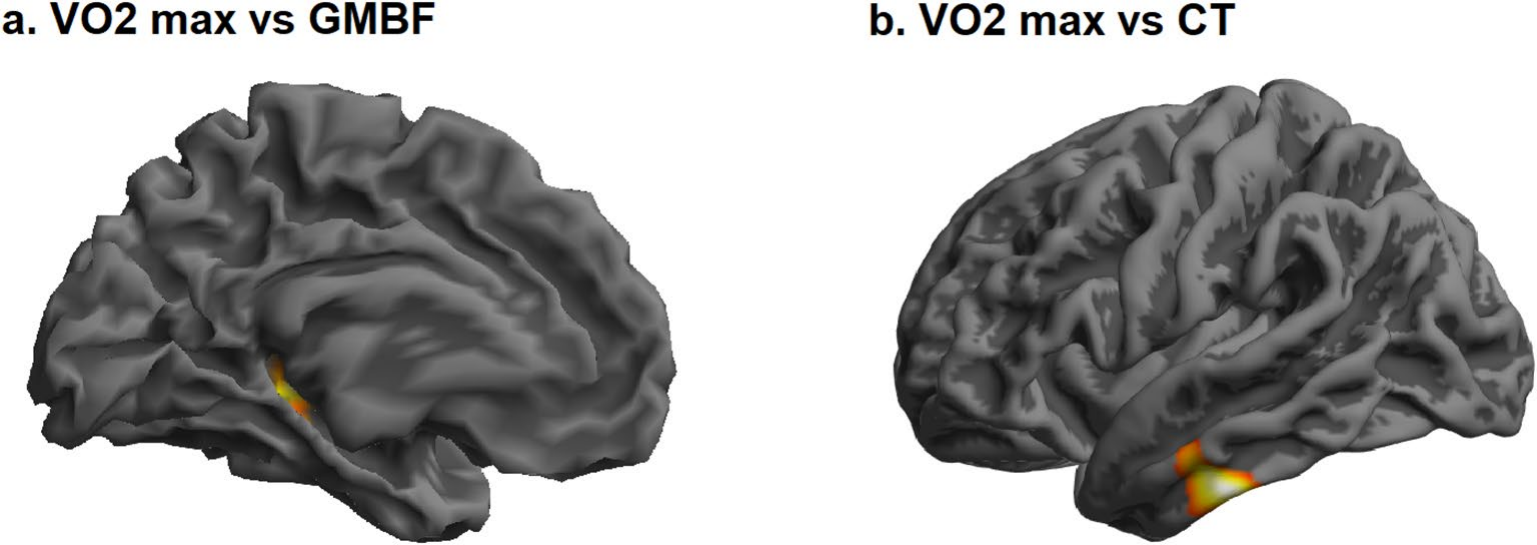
Association between VO_2_max and brain measures. The figure shows the clusters were a statistically significant association was detected between VO_2_max and (a) GMBF and (b) CT, respectively. (a) VO_2_max was negatively associated with GMBF of the left hippocampus (p FEW-corr = .0003, t = 5.64, MNI coordinates (x, y, z): −36, −34, 4). (b) VO_2_max was, on the other hand, positively associated with the CT of the left superior temporal gyrus (STG) (p FWE-corr = .006, t = 5.18, MNI coordinates (x, y, z): −56, −21, −3). Clusters were superimposed on a surface rendering in SPM12. The Harvard–Oxford Cortical Structural Atlas was used to derive the location of the coordinates.
